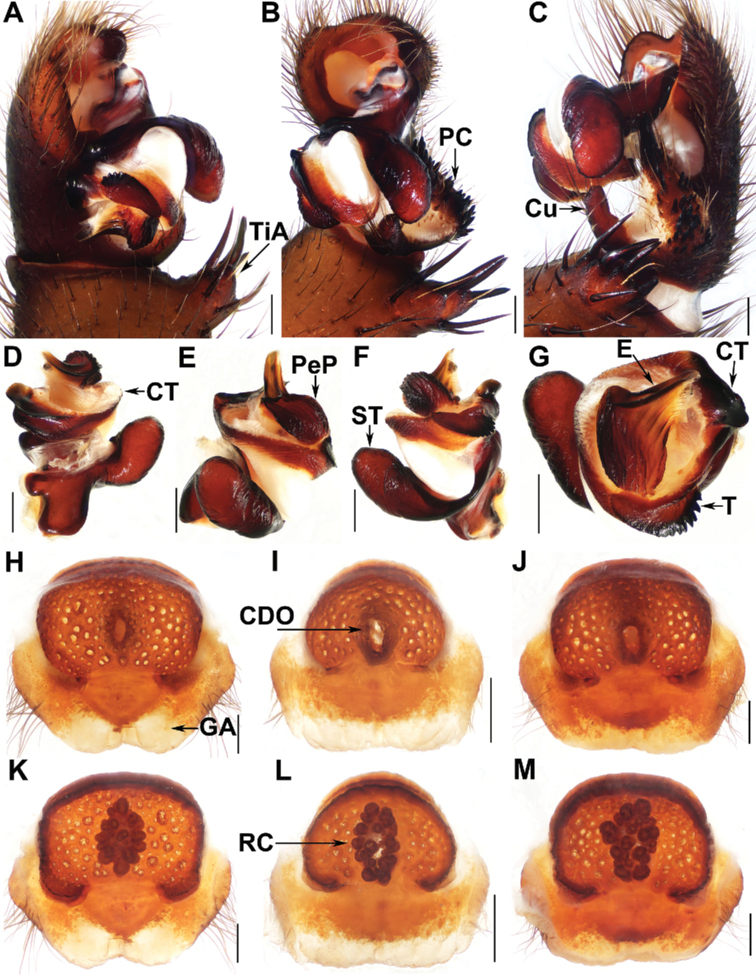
Male palp and female genitalia of *Liphistiusinthanon* Zhan & Xu, sp. nov. A palp, prolateral view B palp, ventral view C palp, retrolateral view D–G palp, distal views H–J vulva, dorsal view K–M vulva, ventral view A–C XUX-2017-372A (holotype) D–G XUX-2017-373A H, K XUX-2017-372 I, L XUX-2017-379 J, M XUX-2017-381. Abbreviations used: CDO = central dorsal opening; GA = genital atrium; RC = receptacular cluster; CT = contrategulum; Cu = cumulus; E = embolus; PC = paracymbium; PeP = paraembolic plate; ST = subtegulum; T = tegulum; TiA = tibial apophysis. Scale bars: 0.5 mm.
